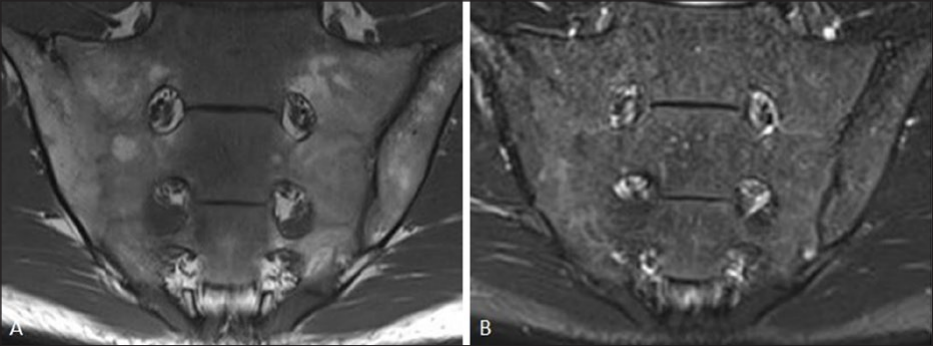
Ankylosis of the SI joint in an 18-year-old boy with JSpA. Semicoronal T1-weighted (a) and STIR (b) images show ankylosis of the right SI joint and narrowing of the left SI joint. No active lesions were seen.
